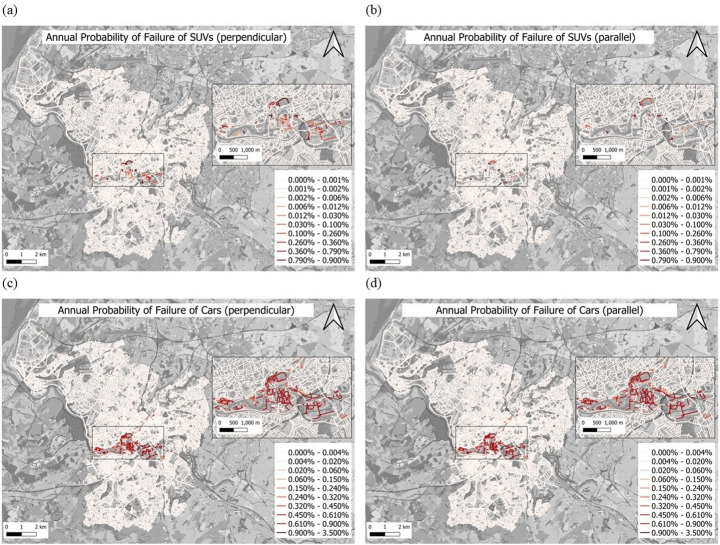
Flood risk maps; annual probability of failure for SUVs a under perpendicular and b under parallel incoming flood; annual probability of failure for cars c under perpendicular and d parallel incoming flood
